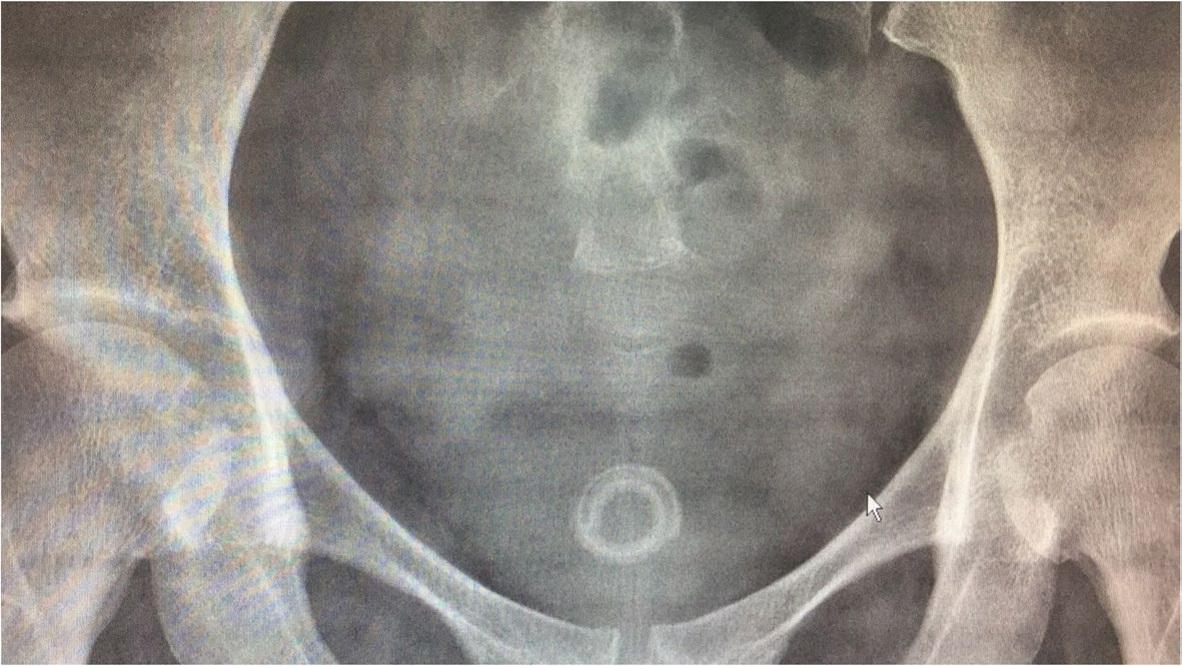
Plain kidney–ureter–bladder (KUB) radiograph demonstrated a high density foreign body in bladder
